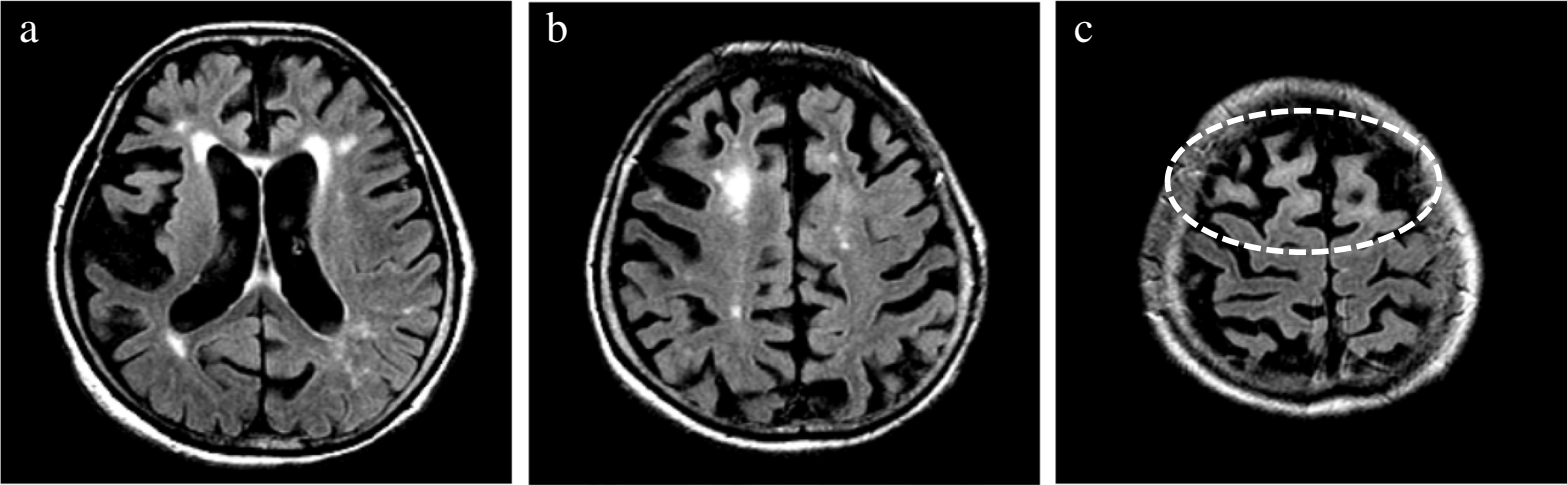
Evaluation of SWMH. The presence/absence of hyperintensity confined to the subcortical white matter (i.e., SWMH) was evaluated on an axial FLAIR image. In contrast to other lobes at lower convexity level (a, b), distinct SWMH of the bilateral frontal lobes at high convexity (c) was observed in a patient with pathologically confirmed CBD. CBD, corticobasal degeneration; SWMH, subcortical white matter hyperintensity
